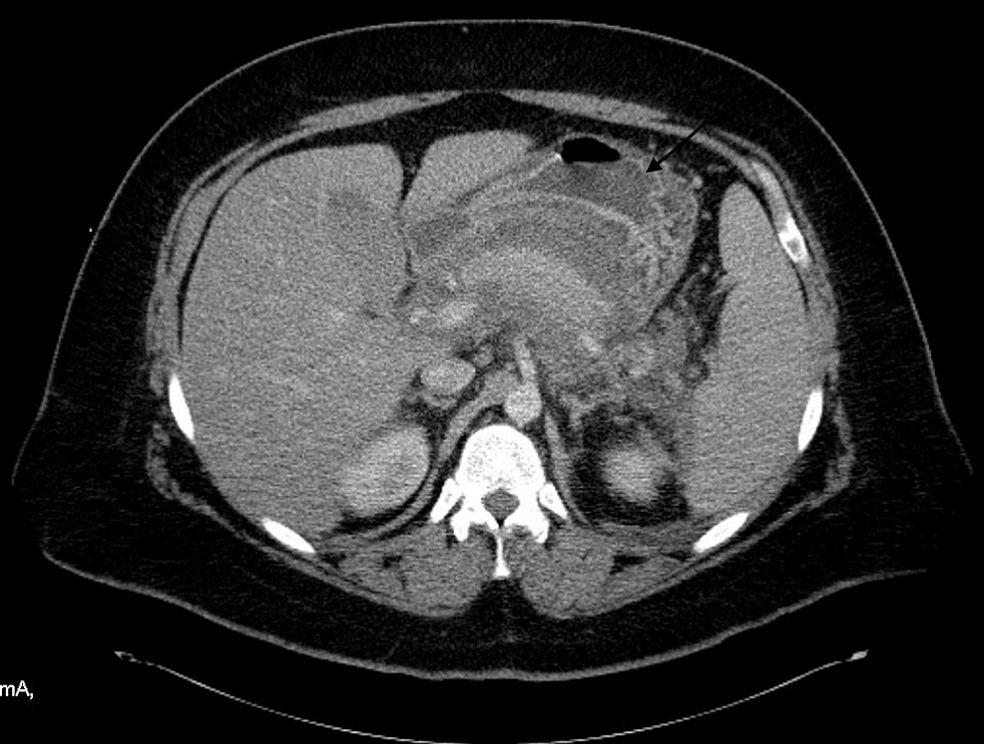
CT scan of the abdomen shows new areas of non-enhancement within the pancreatic tail, compatible with necrosis.
